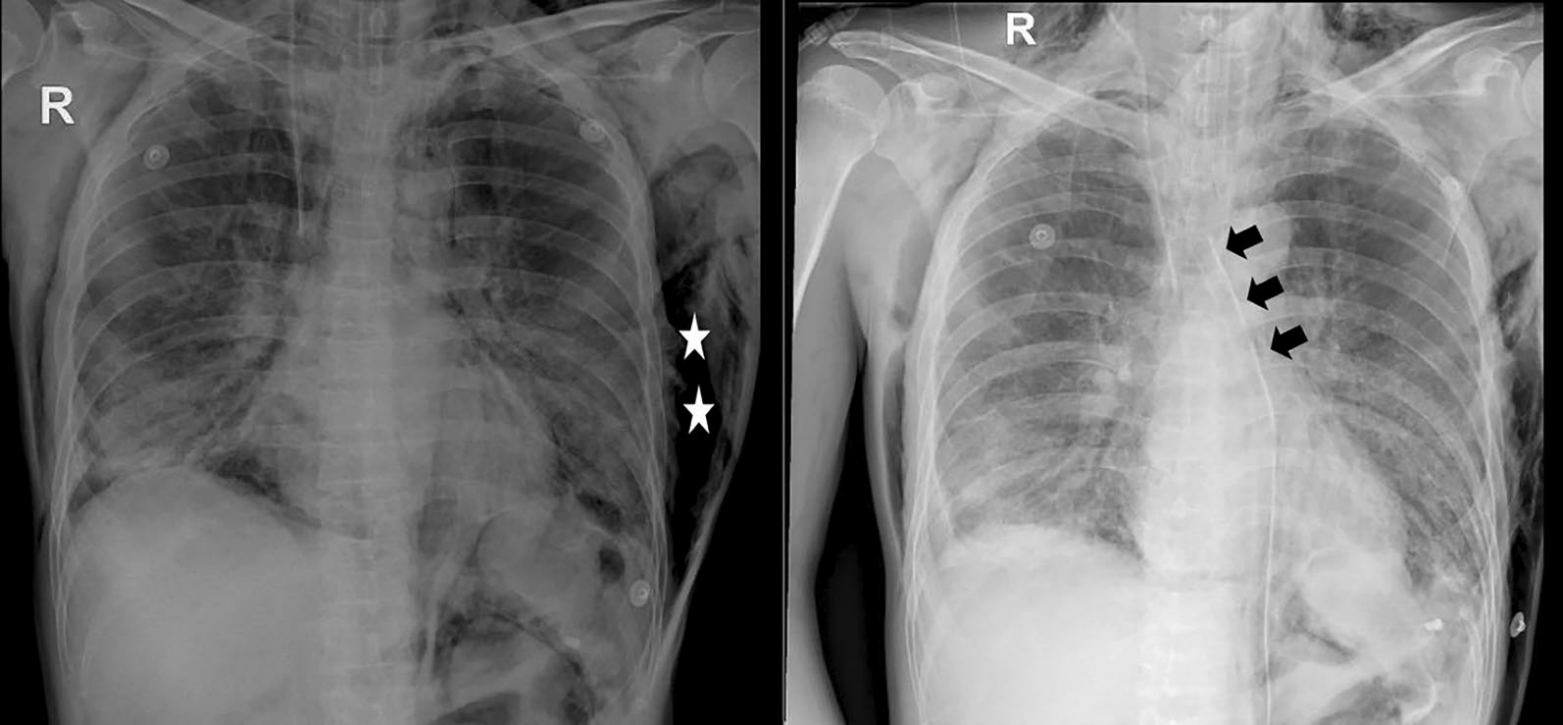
Chest X-ray taken before and after insertion of the drainage tube. Left panel: The chest X-ray taken before drainage shows the presence of remarkable pneumomediastinum. Right panel: The TPM resolved completely after the procedure. The arrowheads indicate the mediastinal drain tube
